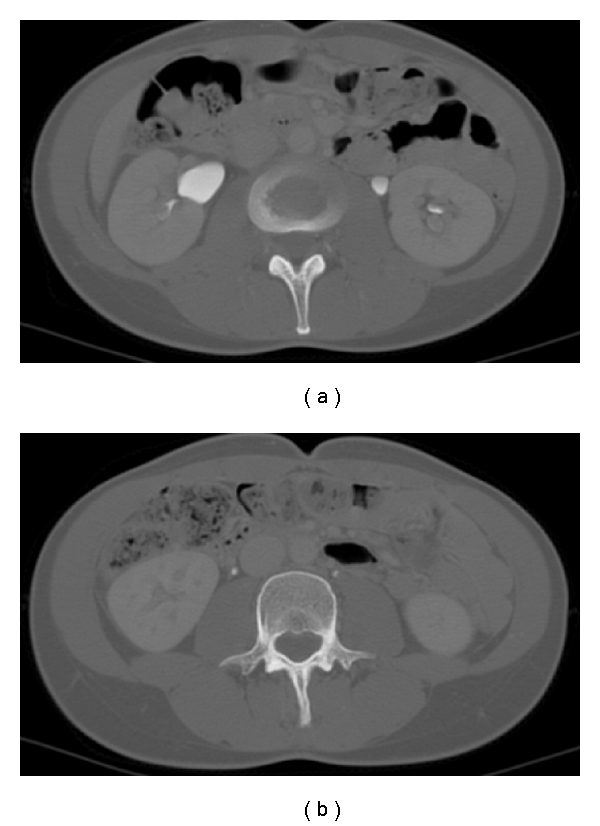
CT evaluation shows the decreasing of the pielic dilation with a good caliber and a normal ureteral morphology.
